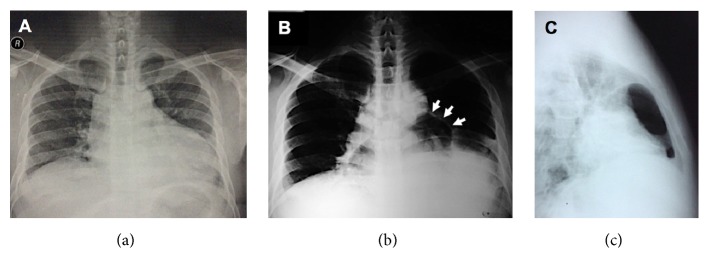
Evolution of the patient's chest radiographs. The prepericardiocentesis film (a) showed cardiomegaly secondary to the massive pericardial effusion. Five days after removal of fluid, new pockets of radiolucency were detected within the cardiac silhouette on both frontal (b) and lateral (c) projections, representing air within the pericardium (arrows pointing at outline of the sac).
